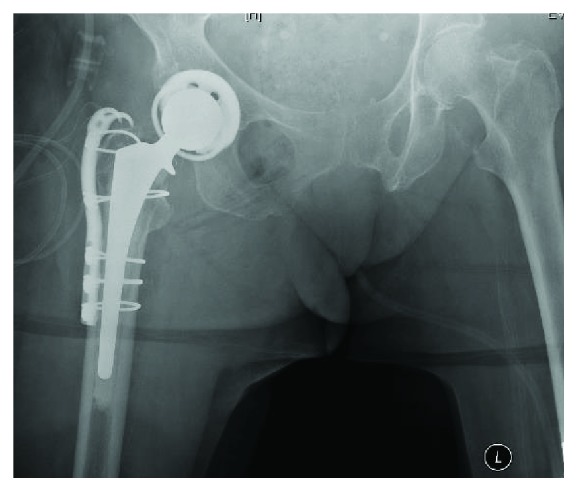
Anteroposterior (AP) pelvis radiograph, status post right hip open reduction, revision total hip arthroplasty with constrained liner and greater trochanteric hook plate with cerclage cables.
